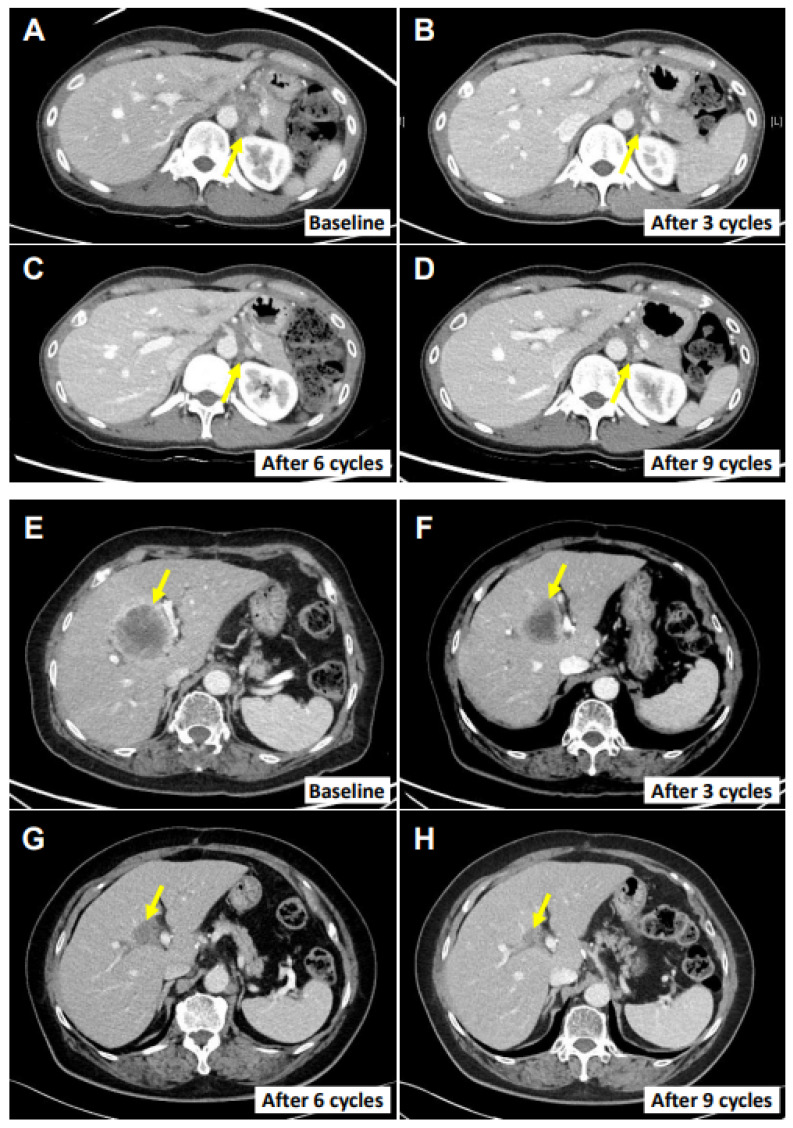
Cancer regression in two patients. CT images of the main tumor lesions at the baseline in each response evaluation. (A–D) Left infrarenal lymph node in patient E0107. (E–H) The main hepatic metastasis lesion in patient E0217. Yellow arrows indicate the target lesions. Response evaluations were performed after every three cycles.
